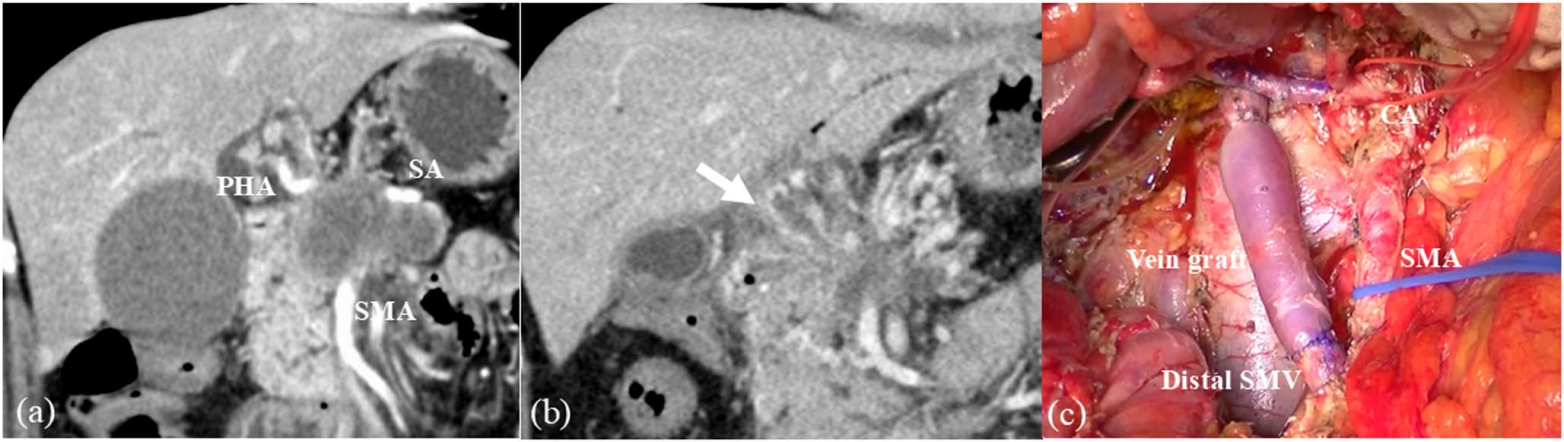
A representative UR-CA & SMA case. (a) Computed tomography shows remarkable cancer invasions around the celiac artery, common hepatic artery, proper hepatic artery (PHA), splenic artery (SA), superior mesenteric artery (SMA), portal vein and superior mesenteric vein. (b) The tumor shrank after the preoperative chemotherapy. However, remarkable collateralization around the pancreas head emerged owing to the occlusion of the portal vein and superior mesenteric vein (arrow). (c) The standardized procedures for the UR-CA case and UR-SMA case were utilized and the patient received pancreaticoduodenectomy with common and proper hepatic arteries resection & reconstruction, splenic artery resection, and portal vein resection & reconstruction using left internal jugular vein graft. Her postoperative course was uneventful, and she remained recurrence-free for postoperative three years
